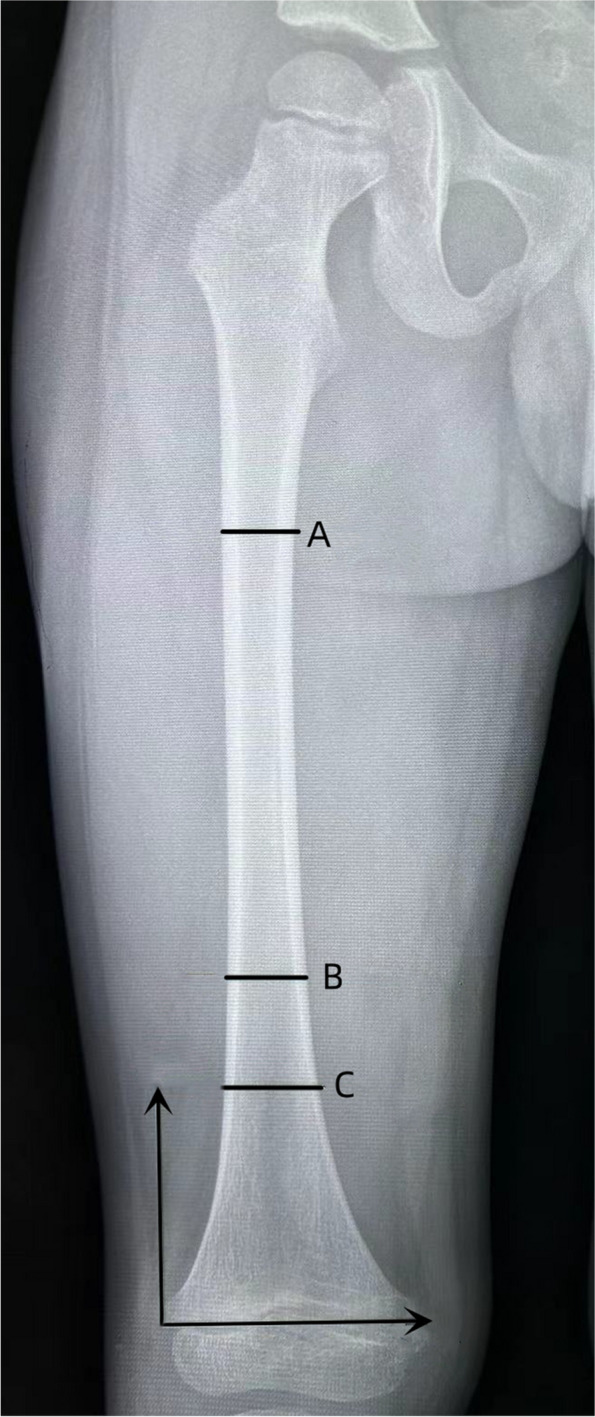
Definition of distal MDJ of femur. Line A and B represent 1/3 border of the proximal and distal femur, and line C represents the proximal border of metaphysis. We defined the MDJ as the femoral segment that lies completely at the proximal end of the distal 1/3 femur. It is bounded proximally by the junctional line between the middle and distal thirds of the femur and distally by the proximal end of the distal femoral metaphyseal region on the anteroposterior (AP) radiograph of the femur (Region line B-C)
